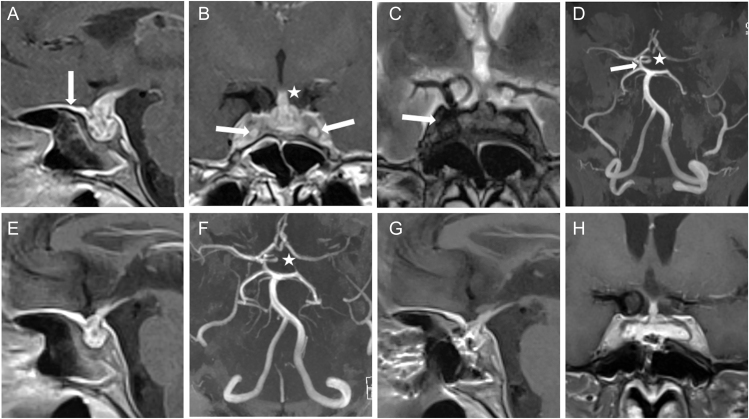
Case 1. (A) Sagittal T1-weighted MRI post-contrast at the time of bilateral ICAo diagnosis in 2015 shows intrasellar hypophysitis with suprasellar extension. Strong dural enhancement is found (arrow). (B) Coronal T1-weighted MRI post-contrast shows bilateral CS infiltration and ICA occlusion (arrows). Non-tapering of the pituitary stalk is found (asterisk). (C) T2-weighted MRI demonstrates parasellar T2 dark sign (arrow). (D) 3D TOF MRA confirms bilateral ICA occlusion (asterisk for right side) and supply of the anterior circulation mainly via a large posterior communicating artery on the left side (arrow). Following treatment with GC and short-term azathioprine, sagittal MRI (Fig. 1E) and TOF MRA (Fig. 1F) from October 2019 demonstrated size reduction of hypophysitis but persistent bilateral occlusion of the ICA. Postoperative sagittal (G) and coronal (H) T1-weighted MRI post-contrast from June 2021 demonstrate a reduced size of the pituitary lesion.
